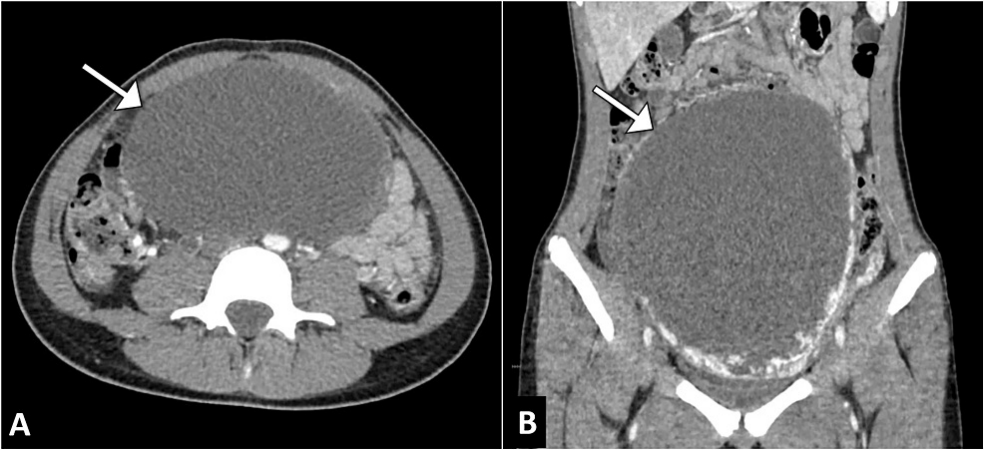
Axial (A) and coronal (B) CT images of the abdomen demonstrating a large unilocular abdominopelvic cystic mass (arrow) causing mass effect by displacing adjacent bowel loops.
